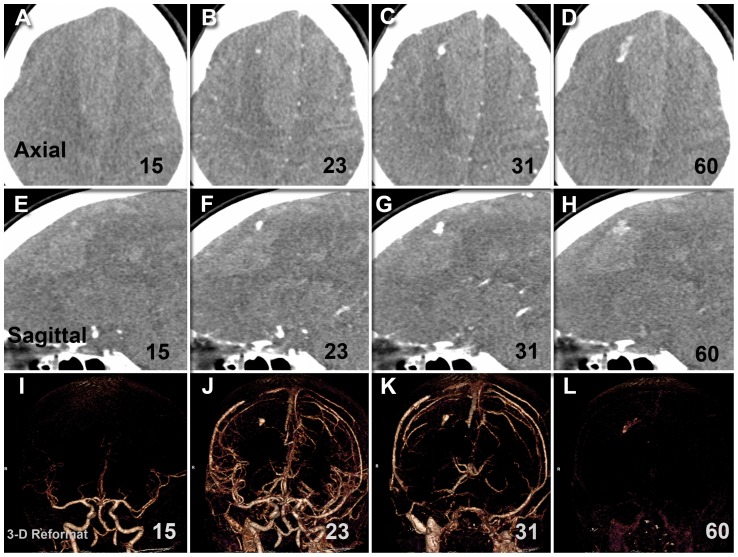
Dynamic CT angiographic image (Axial, sagittal, 3-D reformat) demonstrates the development of a spot sign over the arterial, mid-cycle and venous phases in a subject with large right parasagittal frontal hematoma. It is notable how the spot temporally evolved in its size, shape, volume and density and then eventually faded at the end of venous phase.
